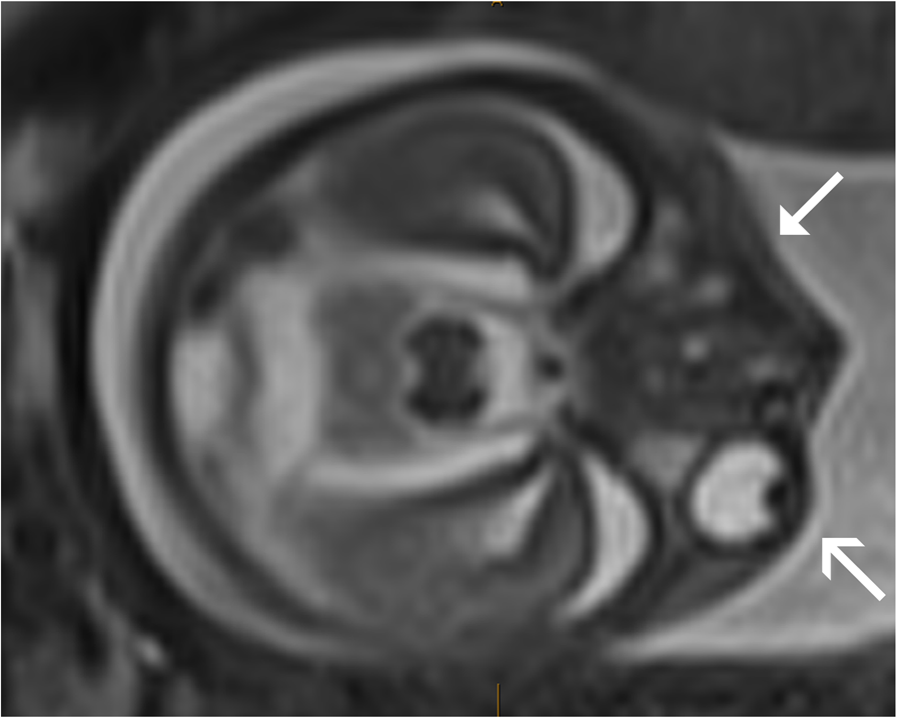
Magnetic resonance images of the fetus with anophthalmia, showing axial views of the fetal head at the level of the eyes. The *triangular arrow-head* represents anophthalmia. The *simple wide arrow-head* represents the normally formed eye
